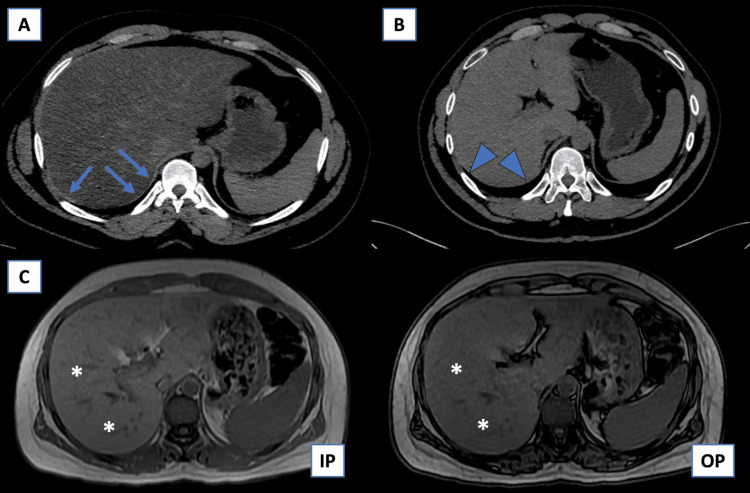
Example of the HRHS in a patient with diffuse fatty liver infiltration (a) NECT scan of a patient with diffuse fatty infiltration of the liver, demonstrating a positive HRHS (arrow), where the diaphragm appears hyperattenuating compared to the adjacent liver parenchyma. (b) NECT scan of a patient with normal liver parenchyma and a negative HRHS, where both the liver and the right hemidiaphragm exhibit similar attenuation and cannot be distinguished (arrowhead). (c) T1 IP (on the left) and OP (on the right) sequences of the same patient in (a), showing signal dropout in the out-phase sequence (asterisk), consistent with diffuse fatty infiltration of the liver. HRHS, hyperdense right hemidiaphragm sign; IP, in-phase; NECT, non-enhanced CT; OP, out-phase
